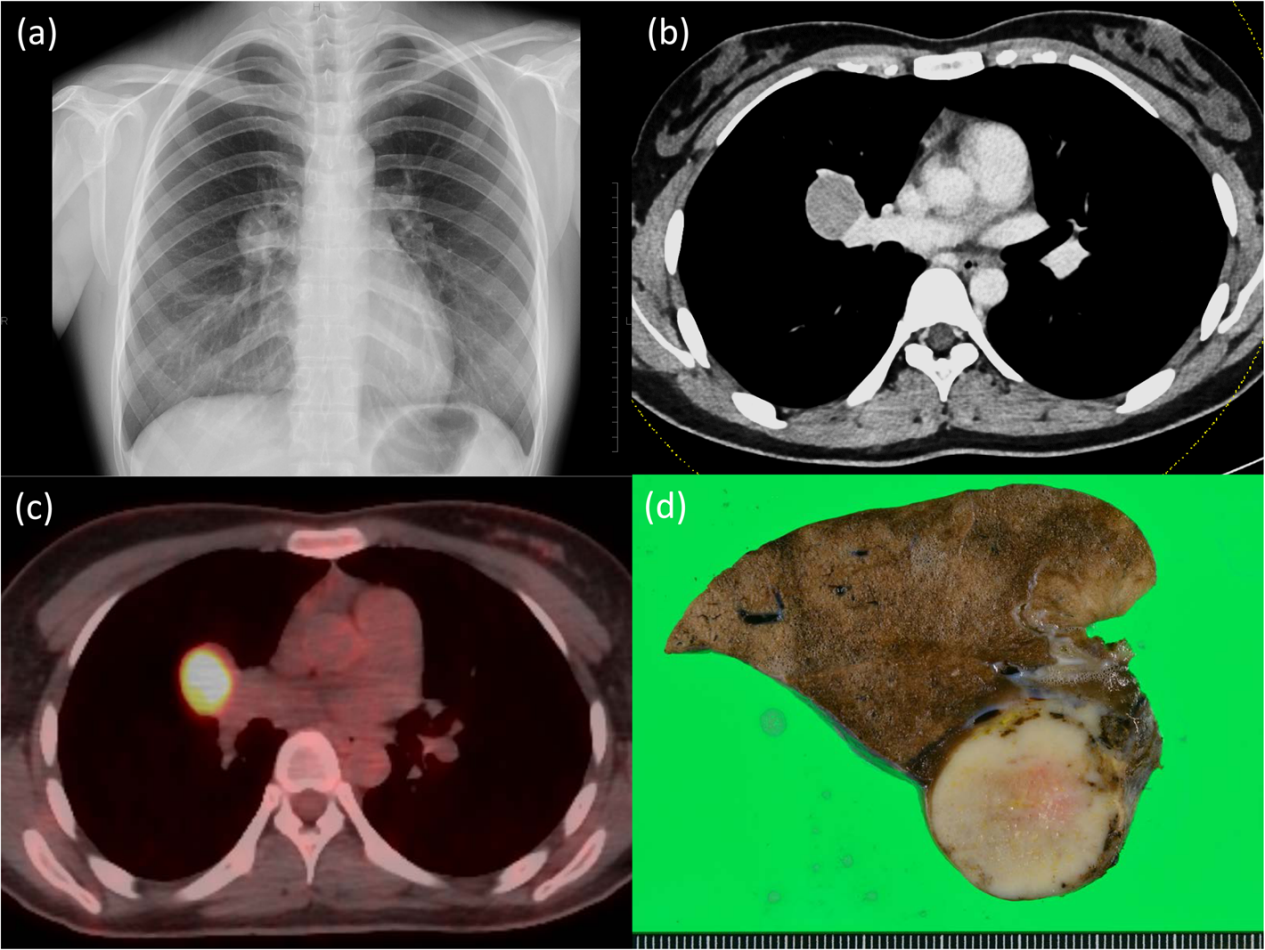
Radiological findings and macroscopic appearance of the tumor. a Chest X-ray revealed a shadow in the right middle lung field. b Computed tomography revealed a tumor in the right upper lobe. c F-18 fluorodeoxyglucose positron emission tomography revealed high accumulation of FDG in the lesion of the right upper lobe. d Macroscopic appearance of the tumor, which appeared as a solitary, white-colored, well-defined, and solid component
